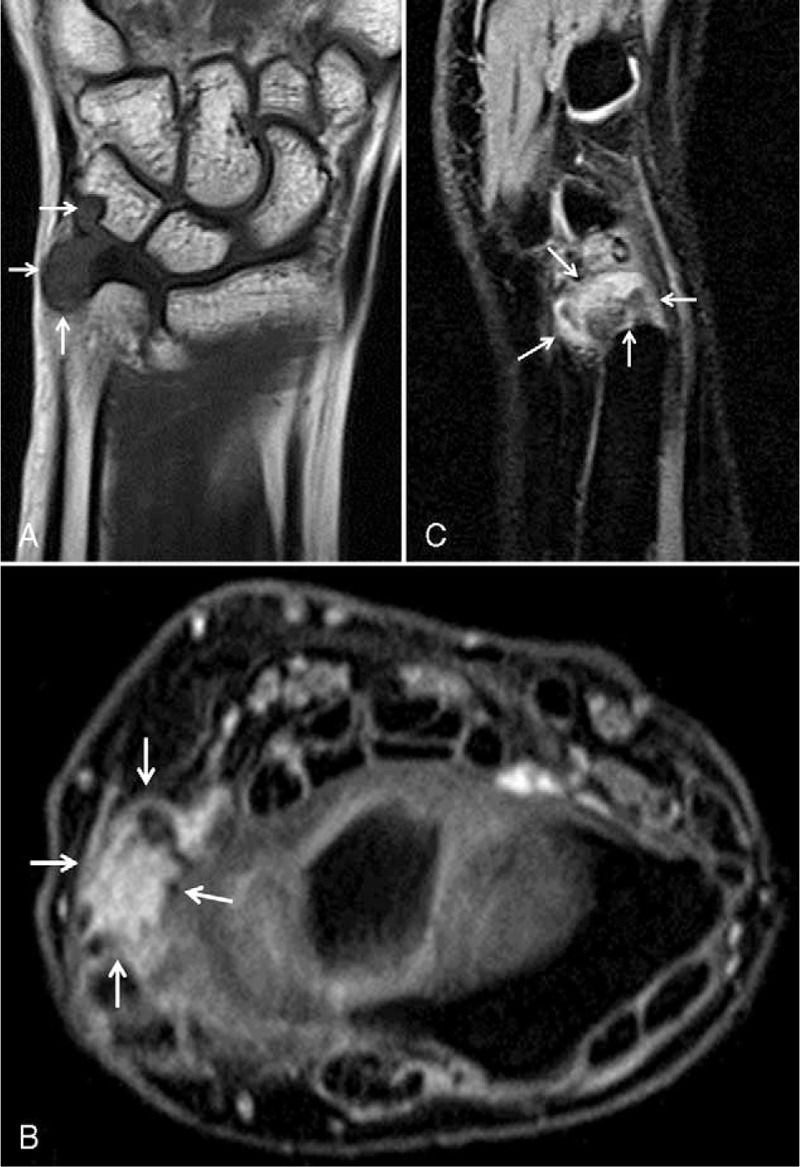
A, Coronal T1-weighted magnetic resonance image of the left wrist, showing a well-defined mass that is slightly hypointense relative to the skeletal muscle and is compressing the triquetrum (arrows). B, Axial fat-suppressed T1-weighted postcontrast. C, Sagittal fat-suppressed T1-weighted postcontrast magnetic resonance images of the left wrist, showing a marked heterogeneous enhancement of the mass (arrows).
